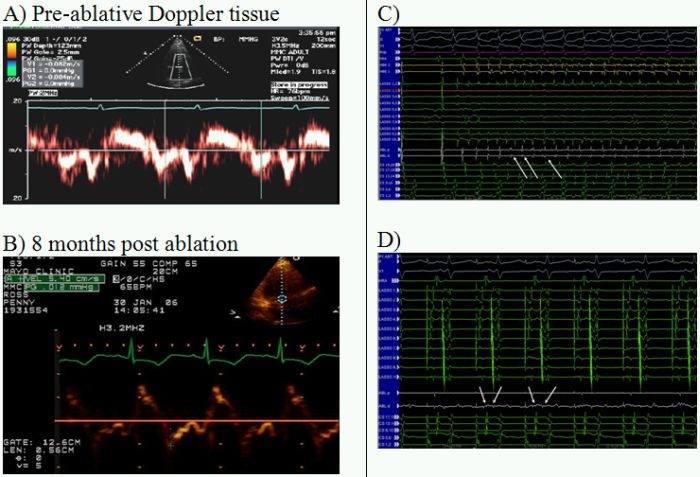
A pre-ablation Doppler velocity tracings at the septal mitral annuls show mild diastolic dysfunction (tissue Doppler e' > 8 cm/s).  Eight months post-ablation (B) showed continued reduced diastolic function (e' 5 cm/s) but corrected volume overload with LAVI decreased from 22 ml/m^2^ from pre-ablation value 33 ml/m2. C/D show pre-ablation electrograms from the left atrium close to the pulmonary vein ostium.  Amplitude is lower and some fragmentation is seen showing early evidence of substrate involvement.
